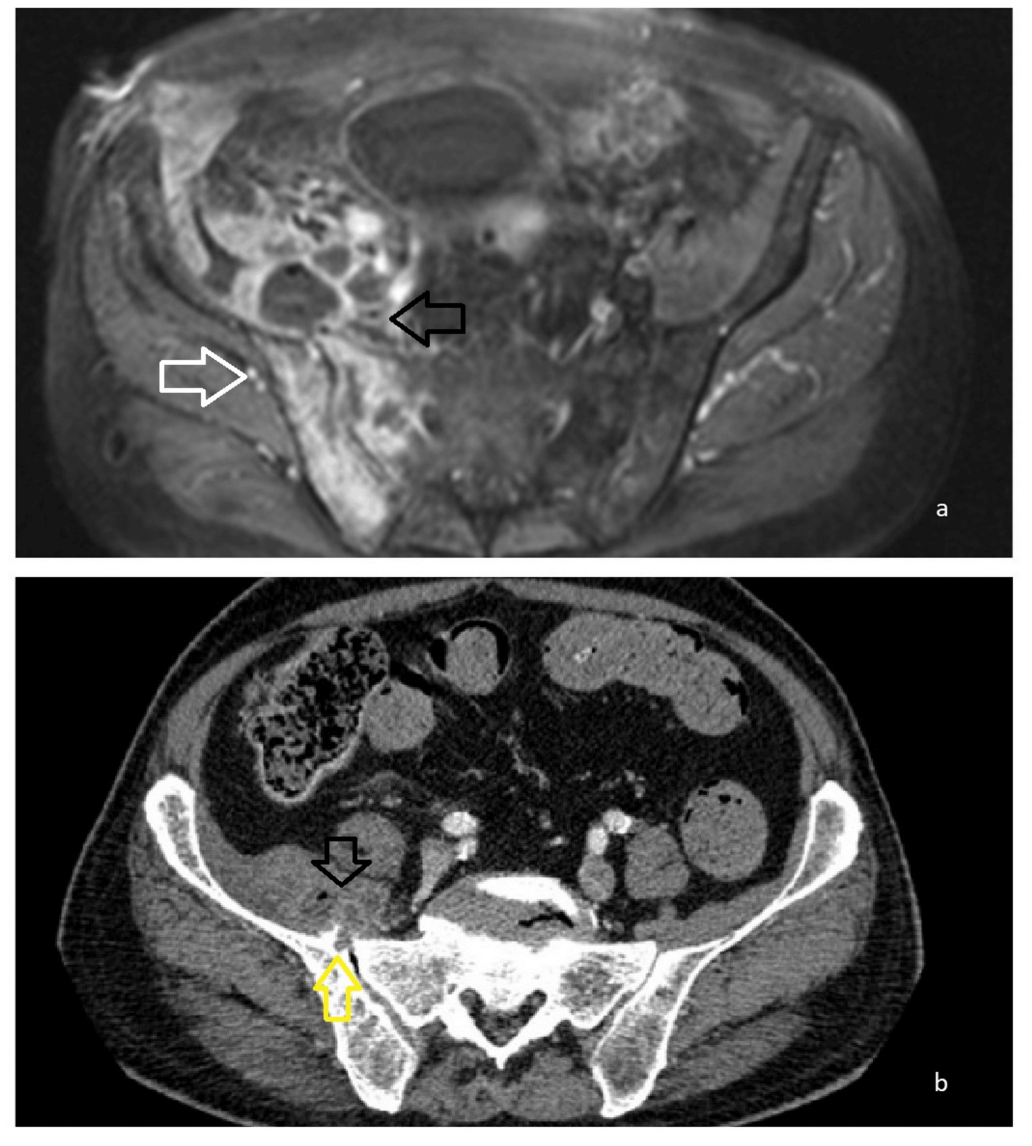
Imaging indicative of deterioration a. MRI of the pelvis T1WI FS +C. Increased and exacerbated enhancement of the right iliac bone and the right sacral wing indicative of osteomyelitis (white arrow). Multiple inflammatory fluid collections of the right iliopsoas muscle of increased size (black arrow). b. Pelvis CT post IV contrast administration. Smaller well-circumscribed inflammatory fluid collections posterior to the right iliopsoas muscle with air bubbles within (black arrow), in contact to the right iliac bone and right sacral wing. Erosion of the right iliac bone (yellow arrow).
